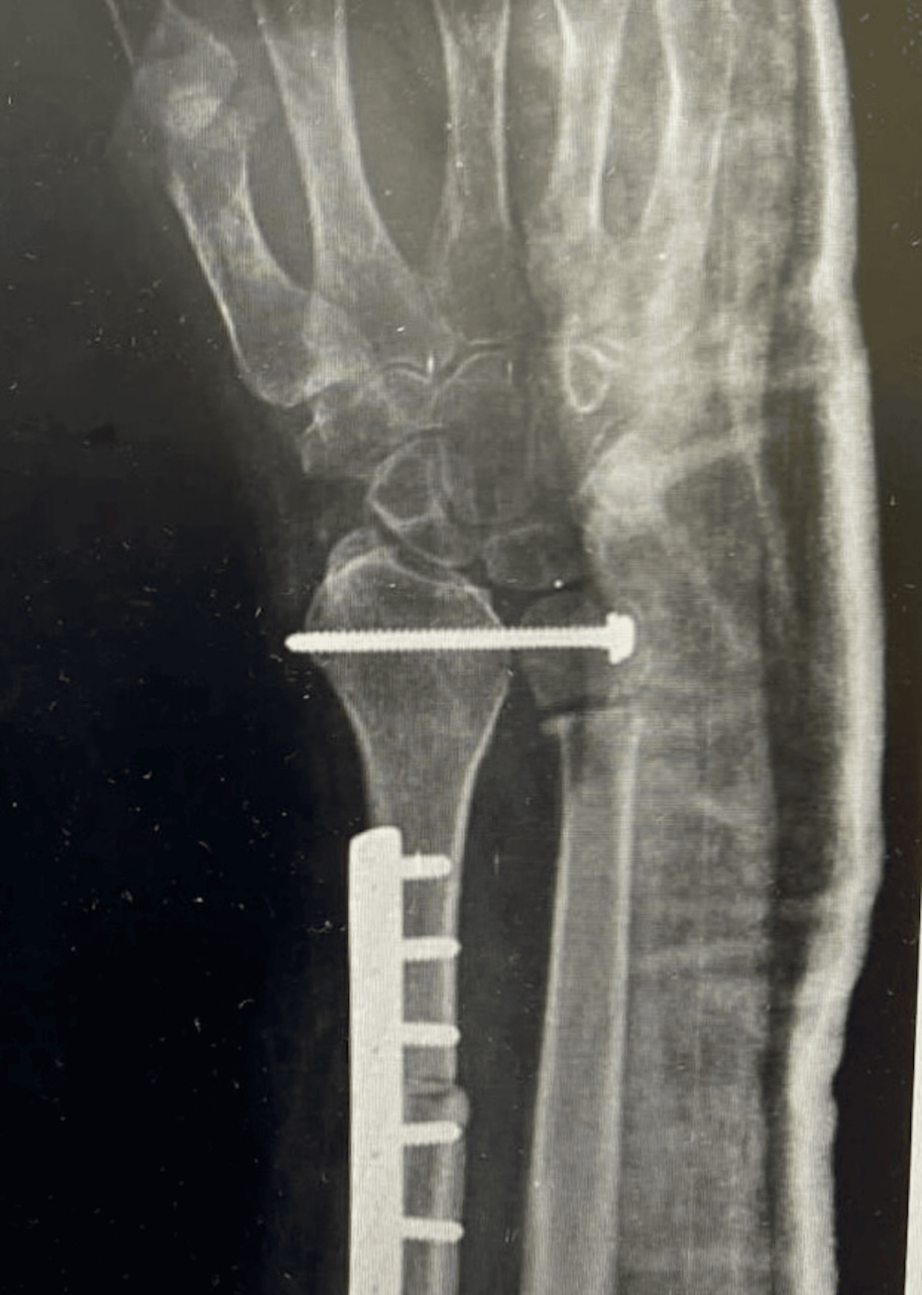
Anteroposterior radiograph of the wrist post-Suavé-Kapandji procedure.
